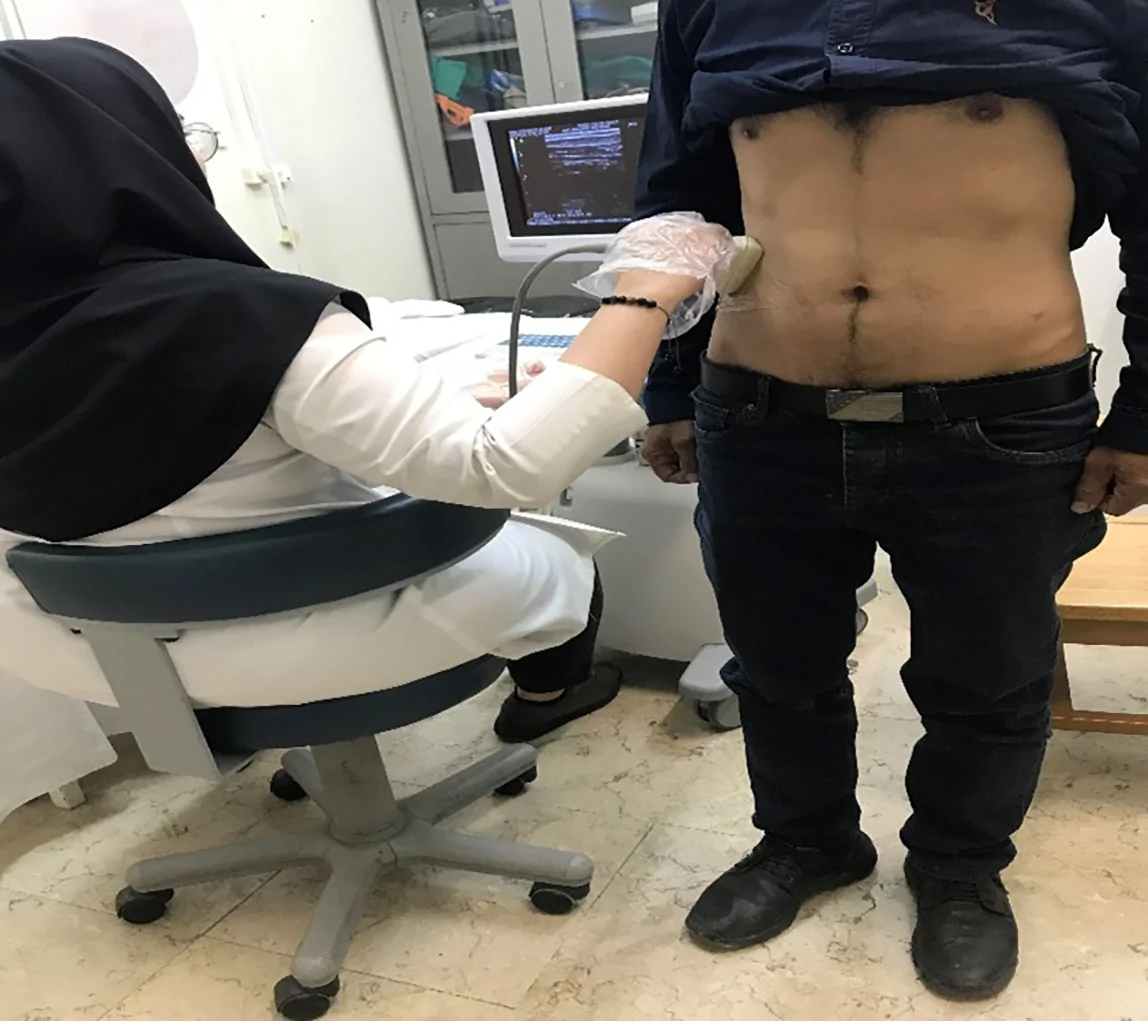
Ultrasound image showing abdominal muscle thickness in the standing position. Created by the authors. Published under CC BY 4.0.
